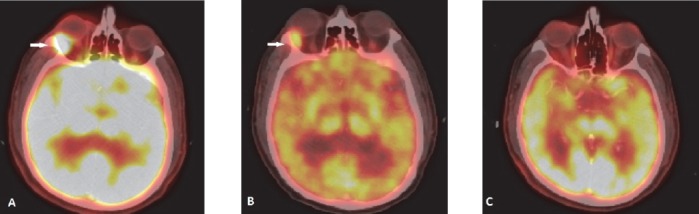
NHL of orbit: (A) FDG avid soft tissue lesion is seen in extraconal compartment of right eye not separable from lateral rectus muscle. (B) Post 3 cycles Bendamustine shows partial metabolic response. (C) Follow up scan after 6 cycles of chemotherapy shows no residual lesion
